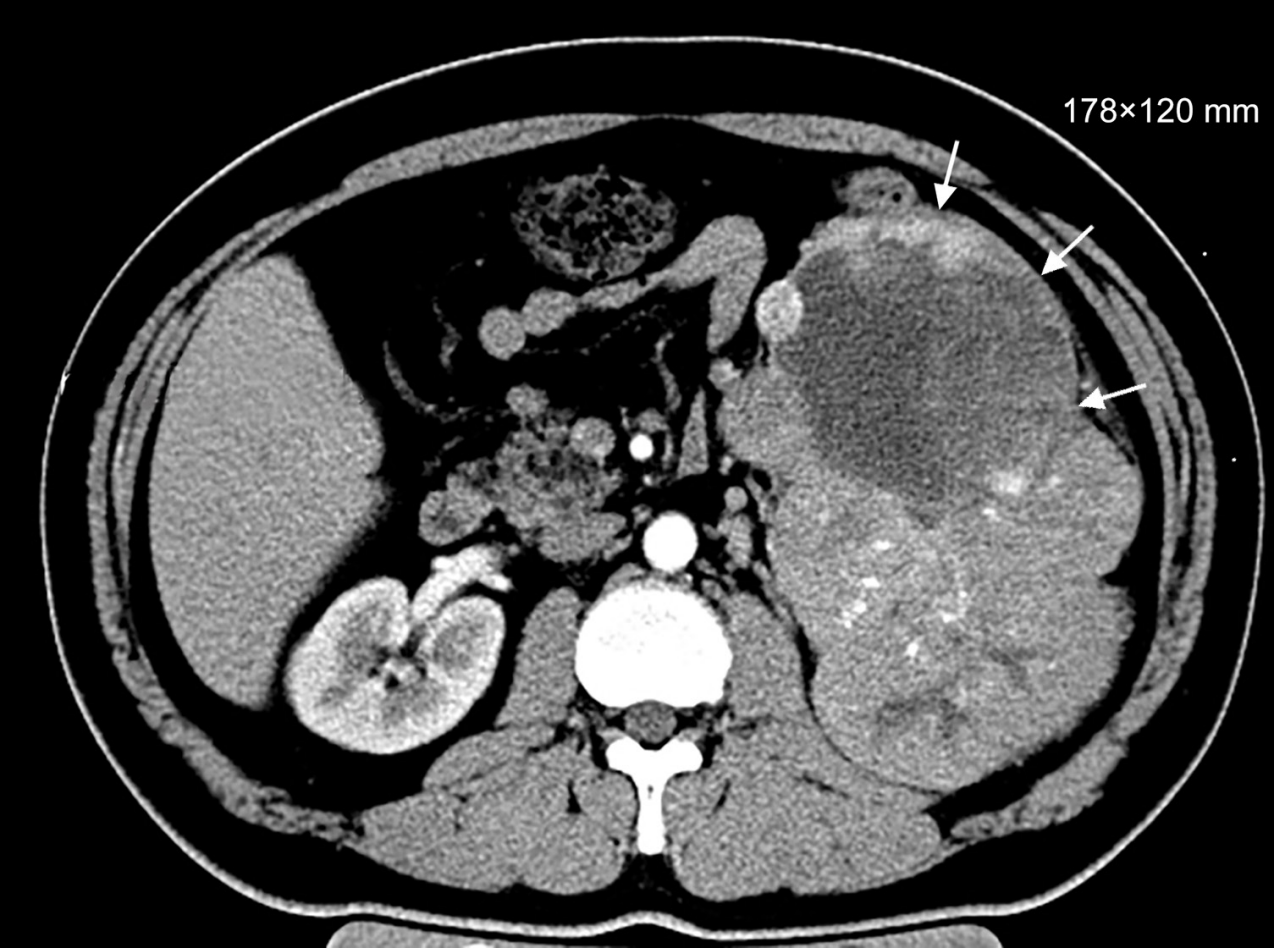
Renal CT (Without any treatment, November 2013)
